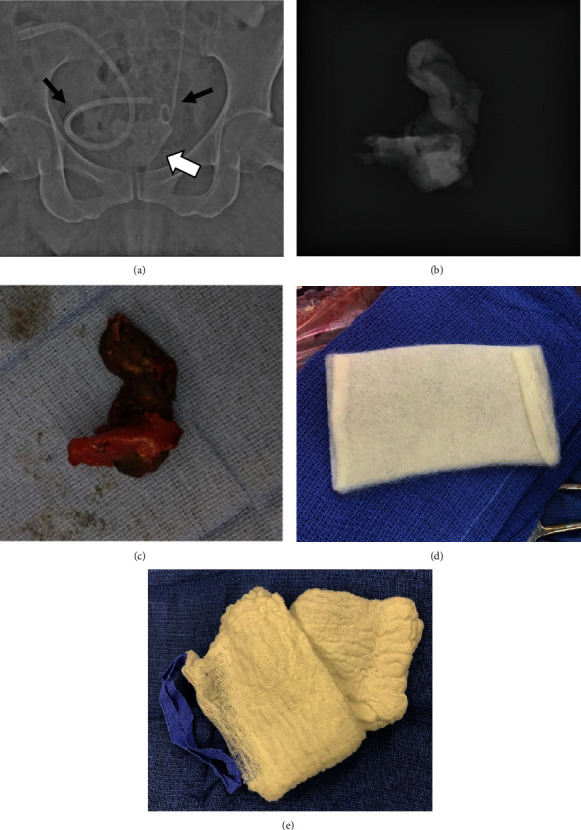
(a) Abdominal radiograph of patient prior to reexploration (white arrow indicates abnormal radiolucency which provoked exploration). Black arrows note the presence of a Jackson-Pratt drain (left) and ureteral stent (right) immediately adjacent to the radiolucent mass of oxidized regenerated cellulose. (b) Radiograph of nonwoven oxidized regenerated cellulose after its removal from the patient. (c) Color photograph of oxidized regenerated cellulose removed from patient's pelvis (1× magnification). (d) Color photograph of fresh oxidized regenerated cellulose (1× magnification). (e) Color photograph of an unused laparotomy pad (1/2× magnification).
